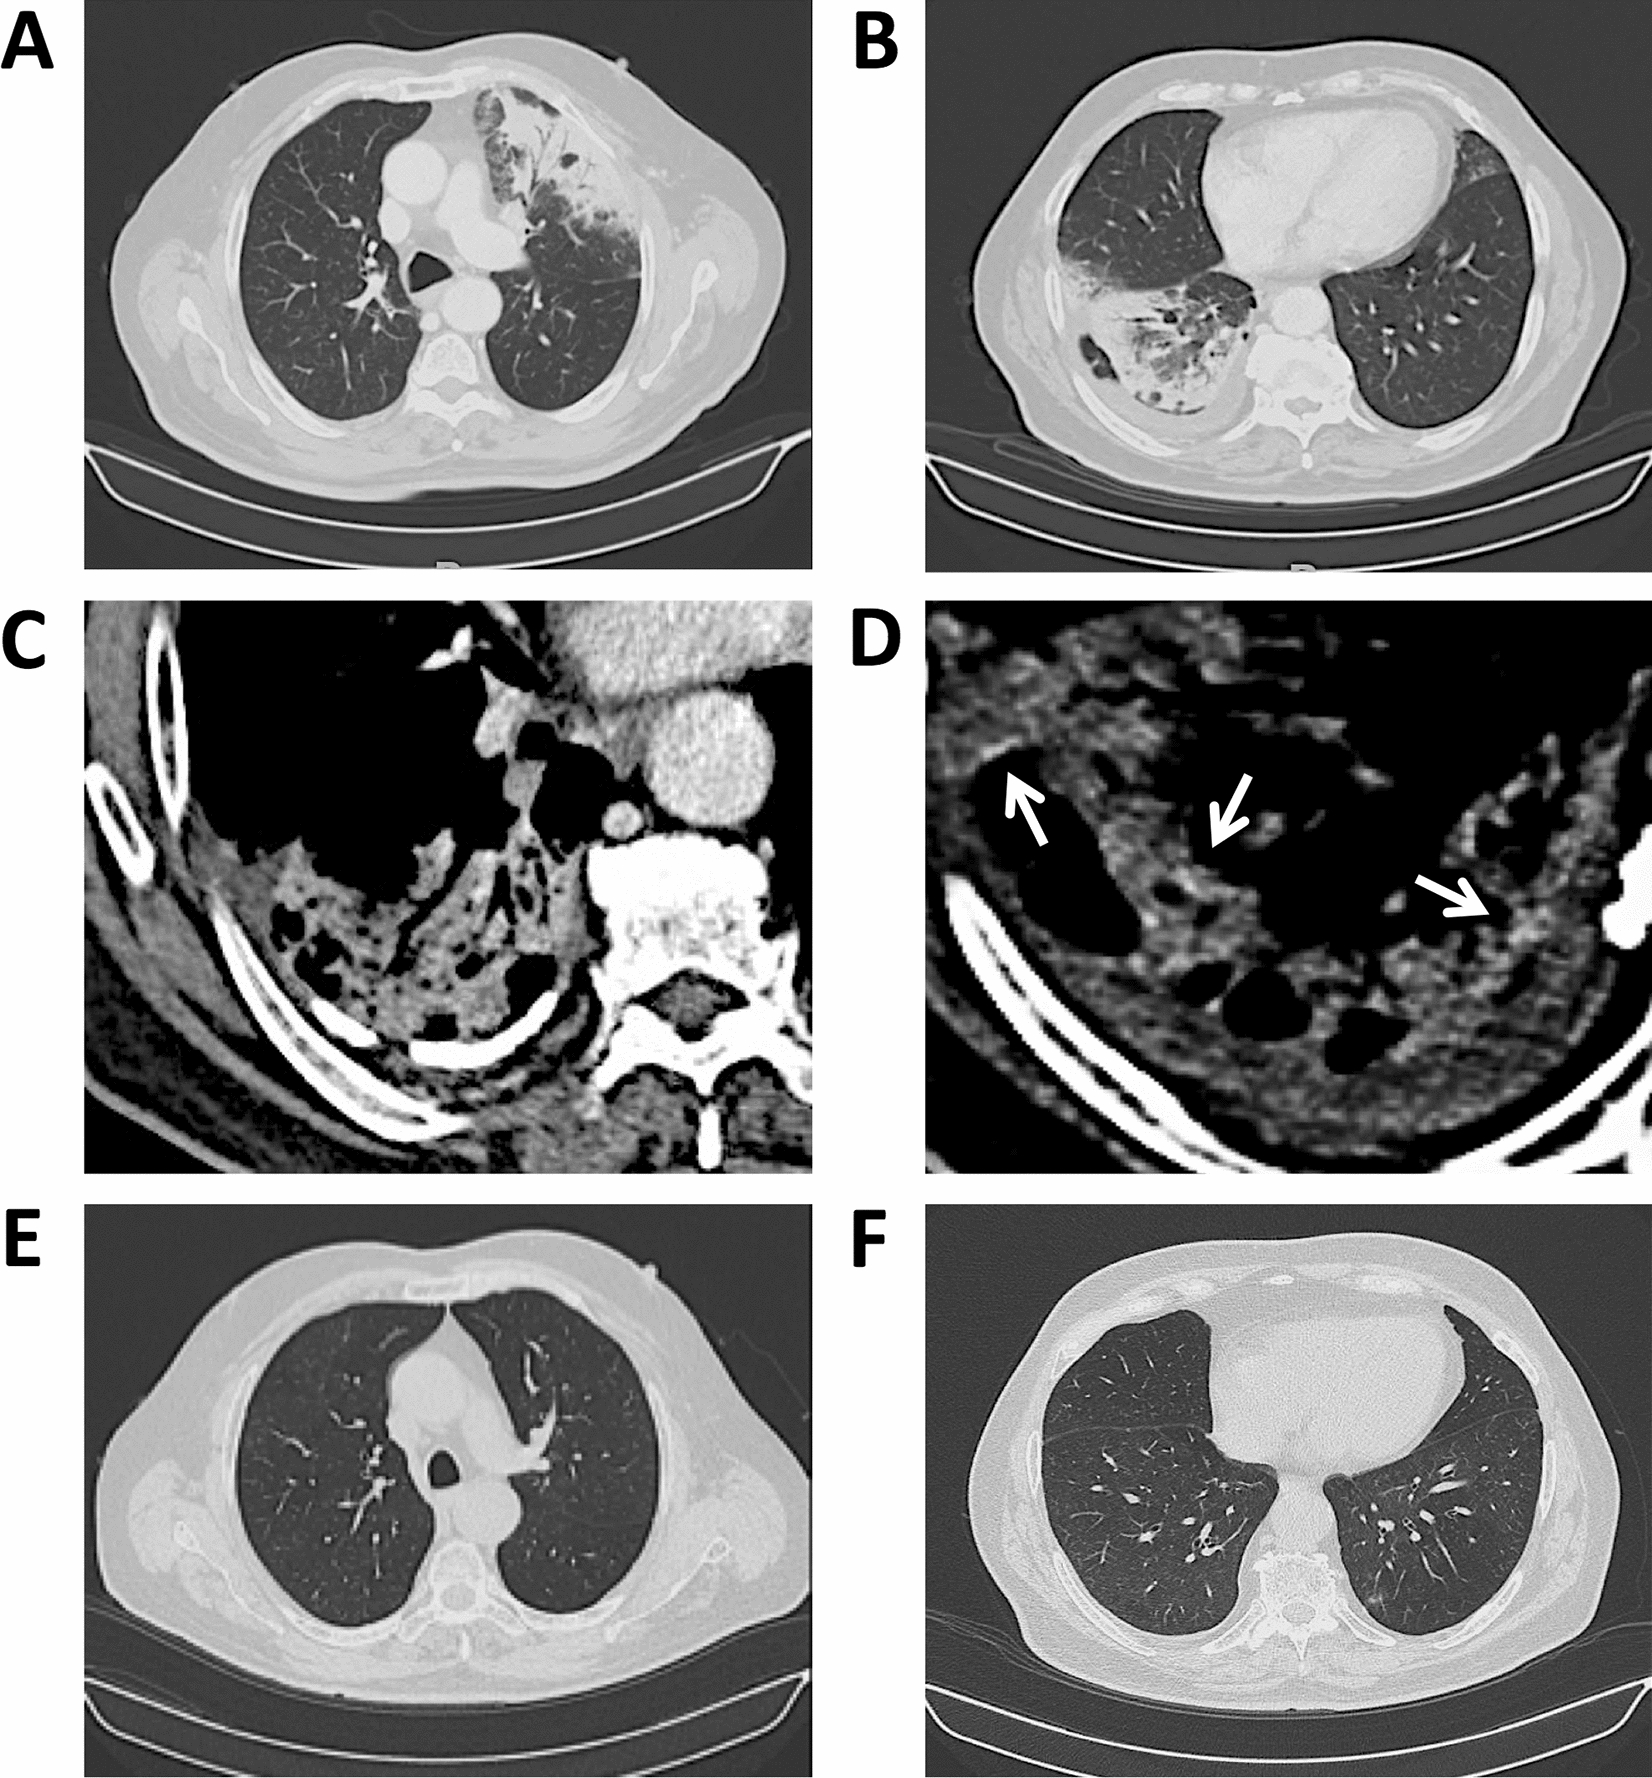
CT scans. A Left upper lobe consolidation with air bronchograms surrounded by ground glass opacities; left hilar lymphadenopathy. B Right lower lobe consolidation delimited by right oblique fissure with air bronchograms; right basal pleural effusion; left anterior pericardial thickening (*lung windows*). C Right posterior pleural thickening and calcification. D Areas of hyperattenuation (110–125 Hounsfield units) (*arrows*) possibly caused by iodine accumulation (*mediastinal windows*). E, F Complete resolution of parenchymal consolidations and pleural effusion following treatment with glucocorticoids and amiodarone wash-out
